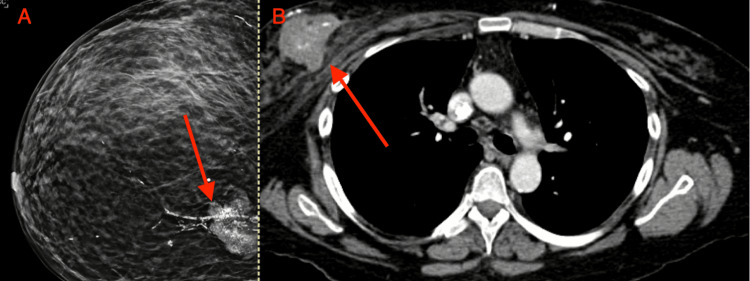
Imaging obtained prior to initiation of neoadjuvant therapy. A. Mammogram demonstrating a right upper quadrant breast mass with pleomorphic calcifications. B. View of right breast mass on CT scan. Red arrow points to the lesion of interest in each image.
